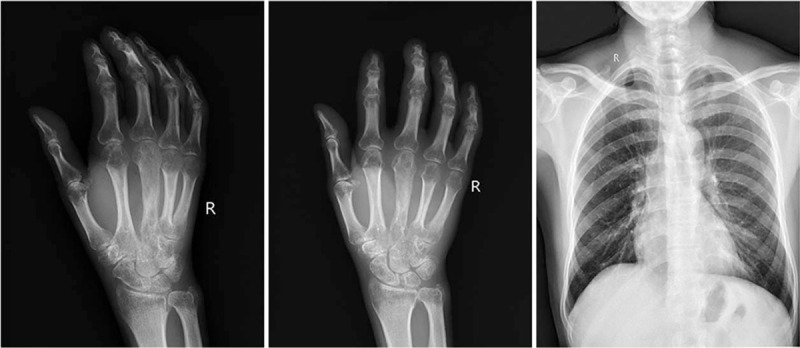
X-ray image before surgery.
